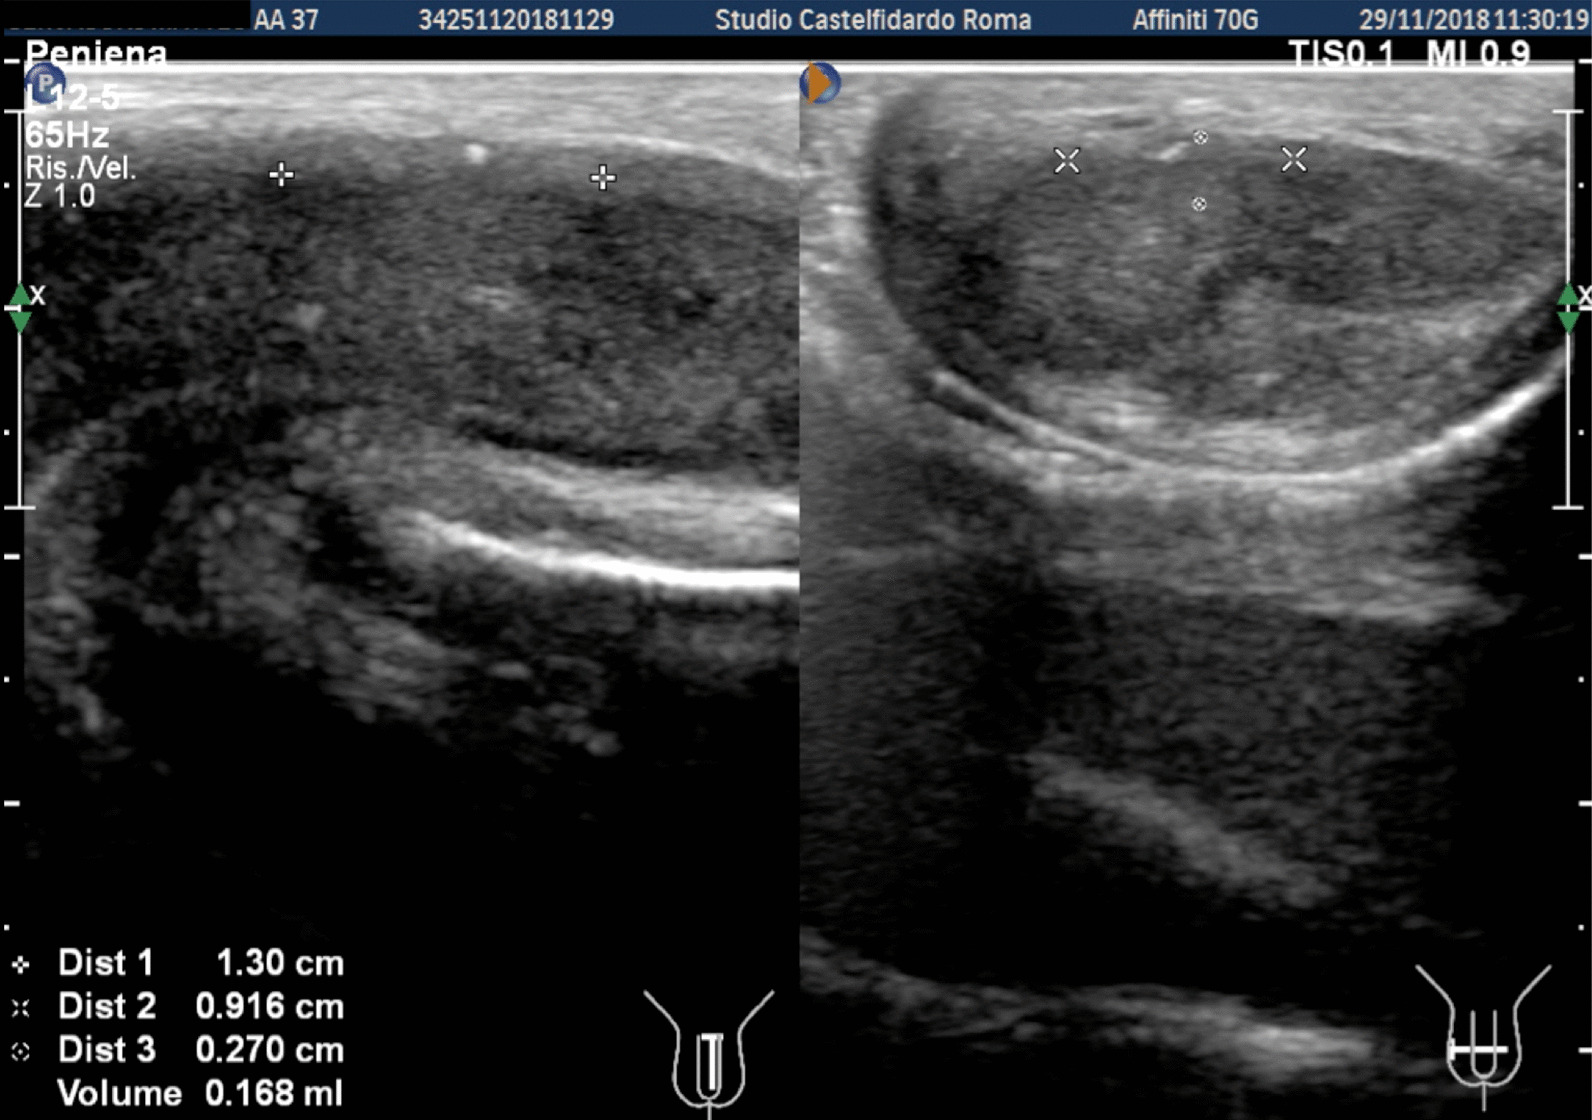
Penile ultrasound after the first treatment cycle (longitudinal and transverse scan)
